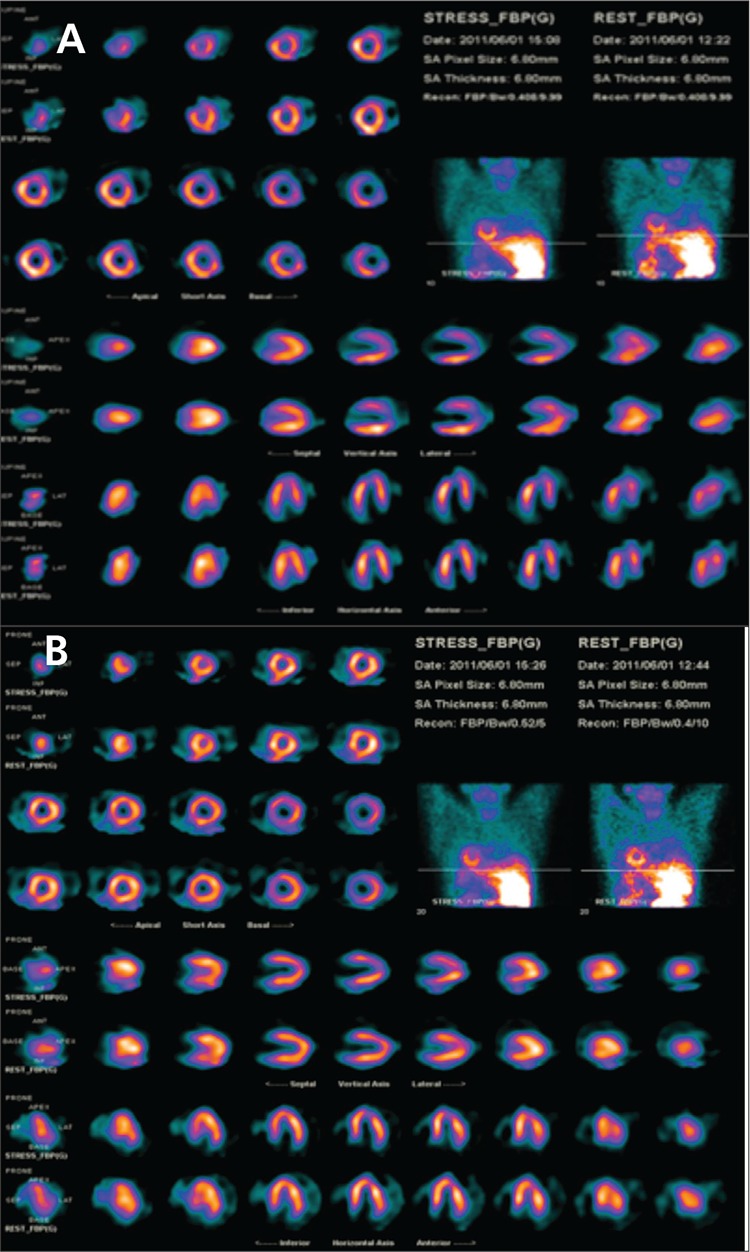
A) Short-axis, vertical long-axis and horizontal long-axis slices and raw data of stress-rest myocardial perfusion SPECT in the normal acqusition and analysis of dextrocardia patient (the septal and lateral walls change locations cause false positive lateral wall defect.).
